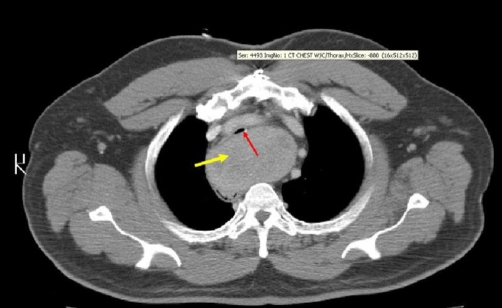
Computed Tomography of the chest showing a 9 cm posterior mediastinal mass (yellow arrow) compromising the tracheal lumen (red arrow).
